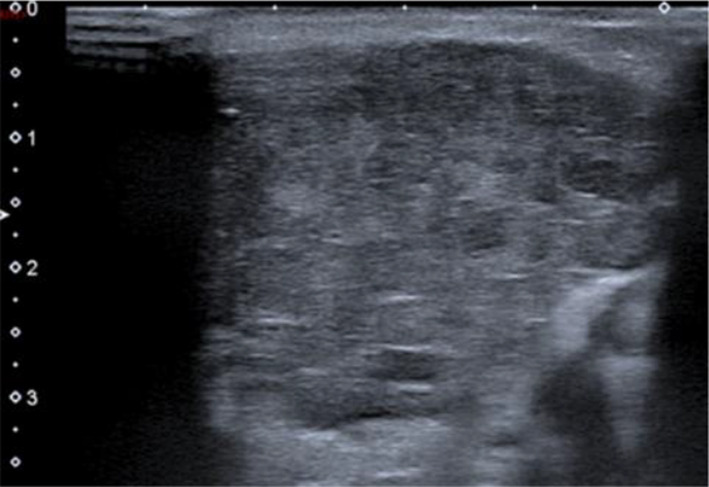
Acute leukemia. Heterogeneous infiltration leading to bilateral parotid gland enlargement, on ultrasonography
